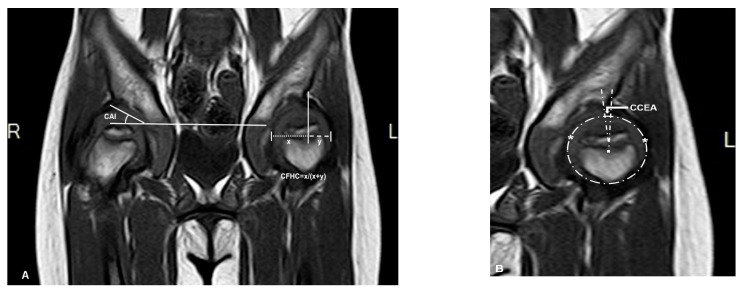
Magnetic resonance imaging (MRI) (coronal T1 sequence) scan of the same patient (patient 3). (A) Right hip measurement of the cartilaginous acetabular index (CAI), left hip measurement of cartilaginous femoral head coverage (CFHC): ‘x’ is the distance between cartilaginous lateral margin of acetabulum and medial margin of femoral head; ‘y’ is the distance between cartilaginous lateral margin of acetabulum and lateral margin of femoral head. (B) Using the method of Siddesh to measure the cartilaginous center-edge angle (CCEA) of the left hip, ‘*’ is the reference points which are marked on the medial and lateral limits of the growth plate. A circle is marked connecting both the reference points ensuring that the circle just touches the inner margin of the epiphysis but does not extend outside the femoral head [22].
